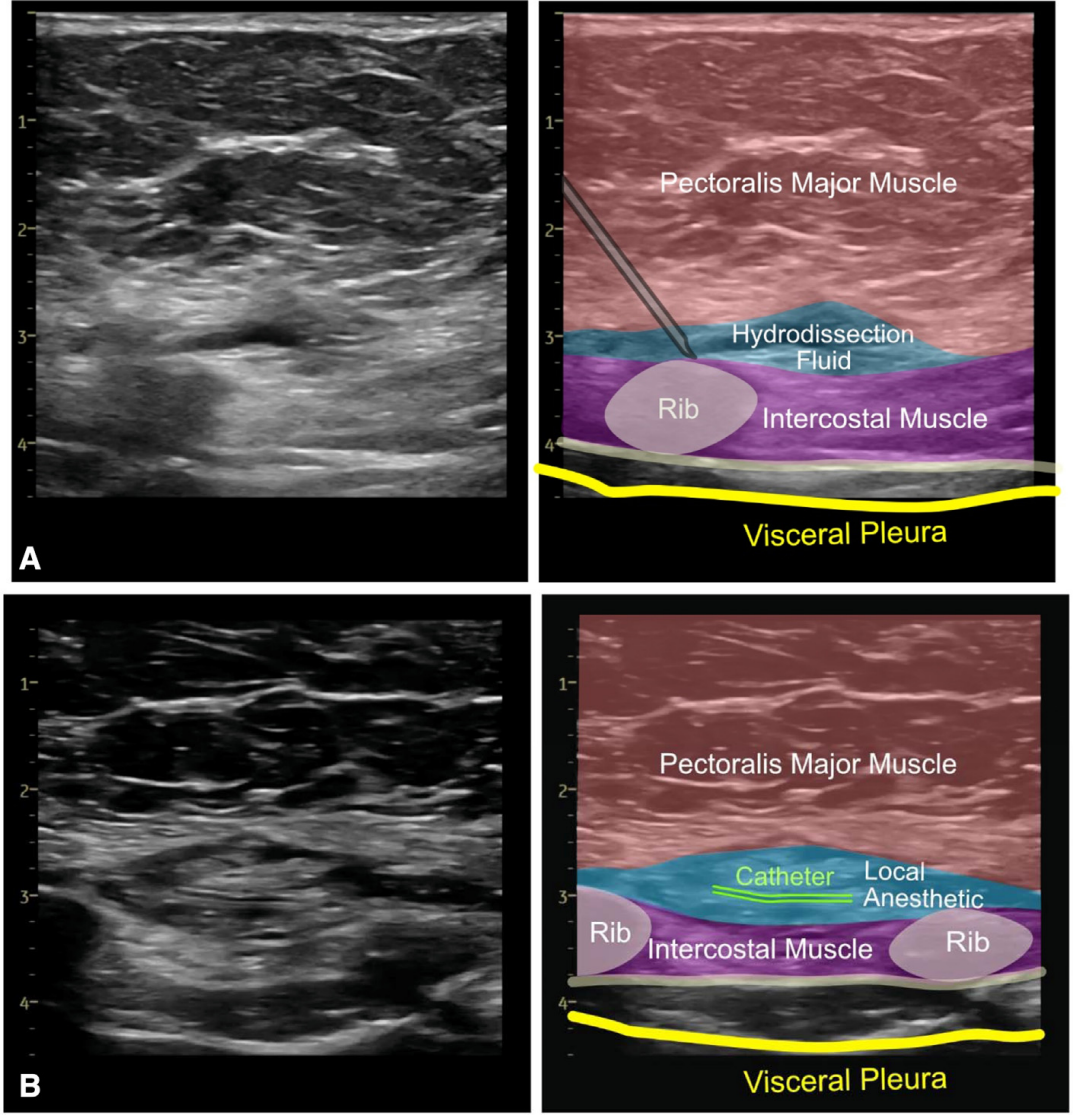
Linear transducer ultrasound images. A, Tuohy needle in the SPIP and spread of hydrodissection fluid. B, Positioning of nerve block catheter in the SPIP. *M*, Muscle; *SPIP*, superficial parasternal intercostal plane.•The needle is then withdrawn, taking care not to dislocate the catheter.•Placement of the catheter tip is visualized by ultrasound. If the catheter is confirmed to be correctly positioned by further “unzippering” of the fascial plane with 1 to 2 mL injectate, LA is injected via the catheter under ultrasound guidance. Regular aspiration attempts are made during the injection to rule out intravascular placement.•The catheter is secured per standard practice with transparent dressings to ensure the entry site remains sterile. We use medical-grade cyanoacrylate glue (eg, Dermabond, Ethicon) to cover the entry points of the catheters and to avoid backflow or leakage of the LA.
